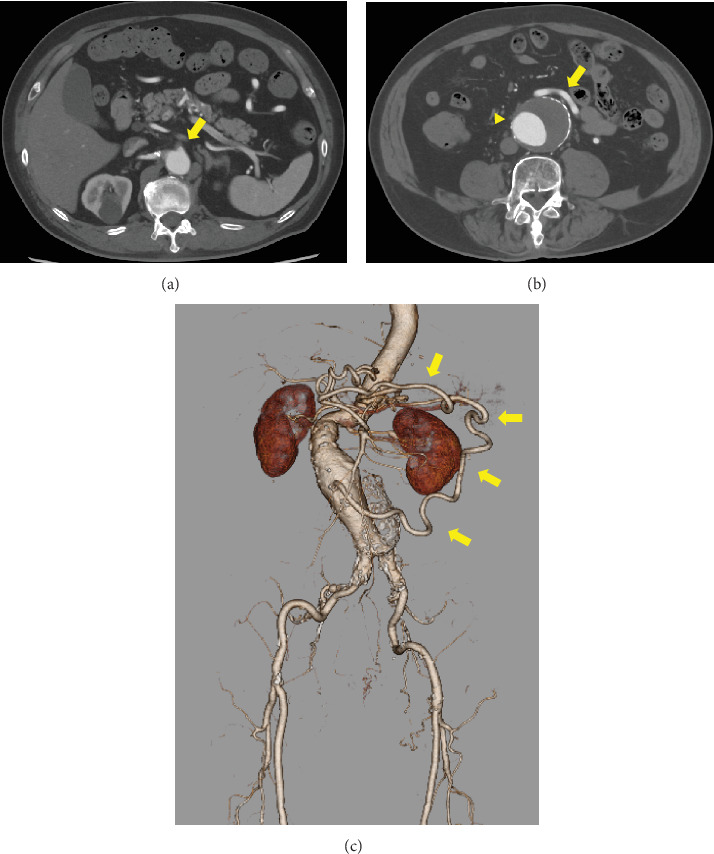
(a) A preoperative contrast-enhanced computed tomography (CT) revealing occlusion of the superior mesenteric artery (arrow). (b) The diameter of the abdominal aortic aneurysm and the inferior mesenteric artery is 53 mm (arrowhead) and 8 mm (arrow), respectively. (c) A multiplanner reconstruction of CT revealing the dilated arch of Riolan (arrows).
